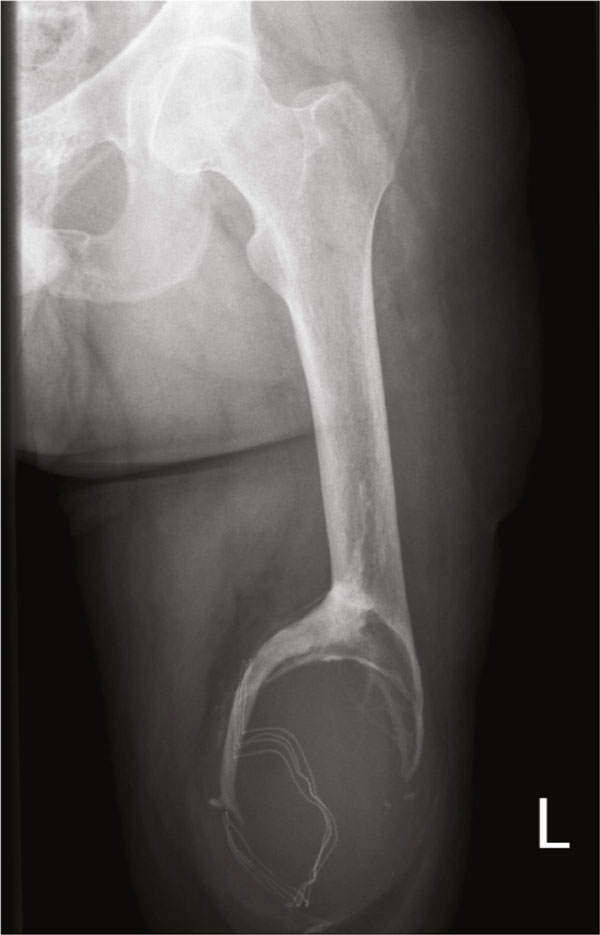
Roentgenogram upper leg with gauze in situ.
